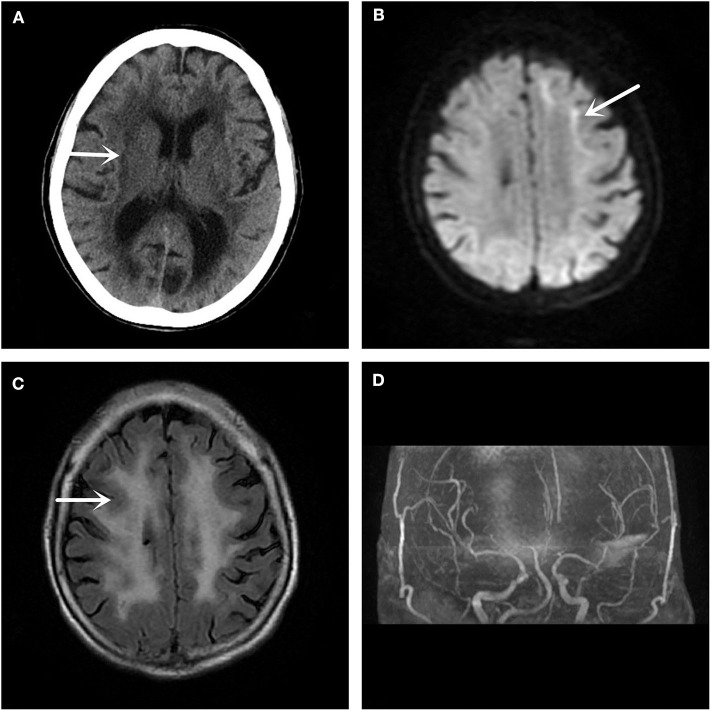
Imaging examination. (A) The head CT shows a low-density lesion in the white matter area. (B) The DWI sequence of the head MRI suggests a high signal shadow in the white matter and gray matter junction area, especially on the left side, which is characteristic of the inclusion disease in the nuclei of the neurons. (C) The T2 Flair sequence of the skull MRI also shows abnormal signals in the white matter region of the brain. (D) No significant intracranial aortic stenosis or occlusion changes are observed in the head MRA.
